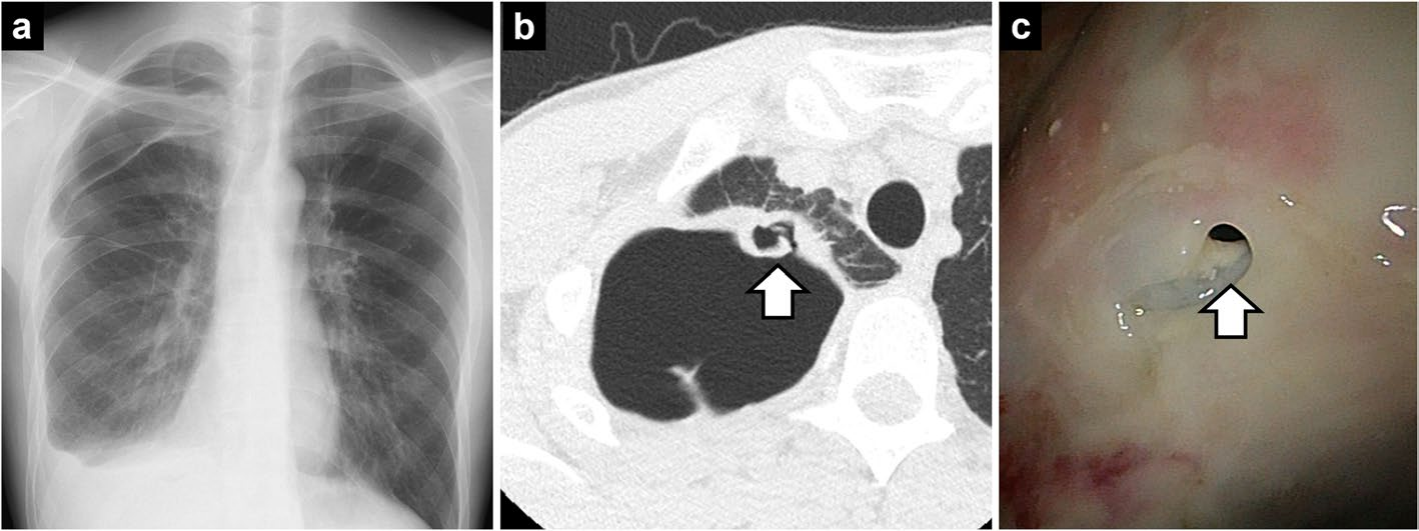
Findings of an aspergillus empyema due to rupture of the cavity of a pulmonary aspergilloma. A Chest radiographs showed a right-sided pneumothorax and pleural fluid. B Computed tomography showed a perforated lung cavity with a suspected aspergilloma in the right apex of the lung (white arrow). C Thoracoscopy revealed a fistula at the apex of the lung (white arrow)
